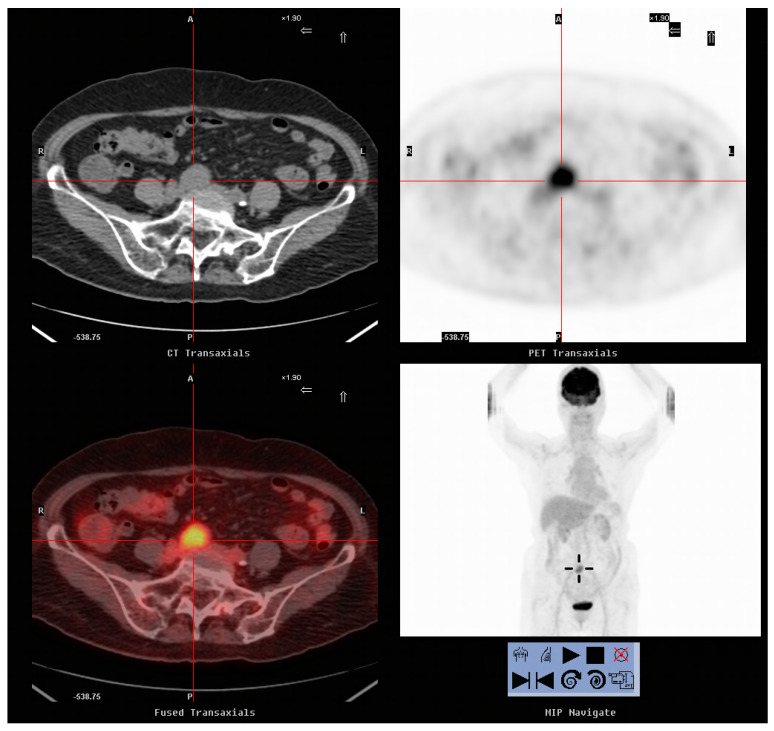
A 78-year-old woman who had cervical cancer (SqCC, carcinoma in situ) 10 years previously presented with the elevation of the serum SCC-Ag level to 6.7 ng/mL. FDG-PET/CT revealed a 3.0 cm FDG-avid nodule over the right pre-sacral area (SUVmax: 5.2, cross-cursor), indicating a recurrent lesion. The patient underwent resection of the nodule, and pathology indicated a metastatic node. Currently, the patient has been disease-free for more than 10 years.
